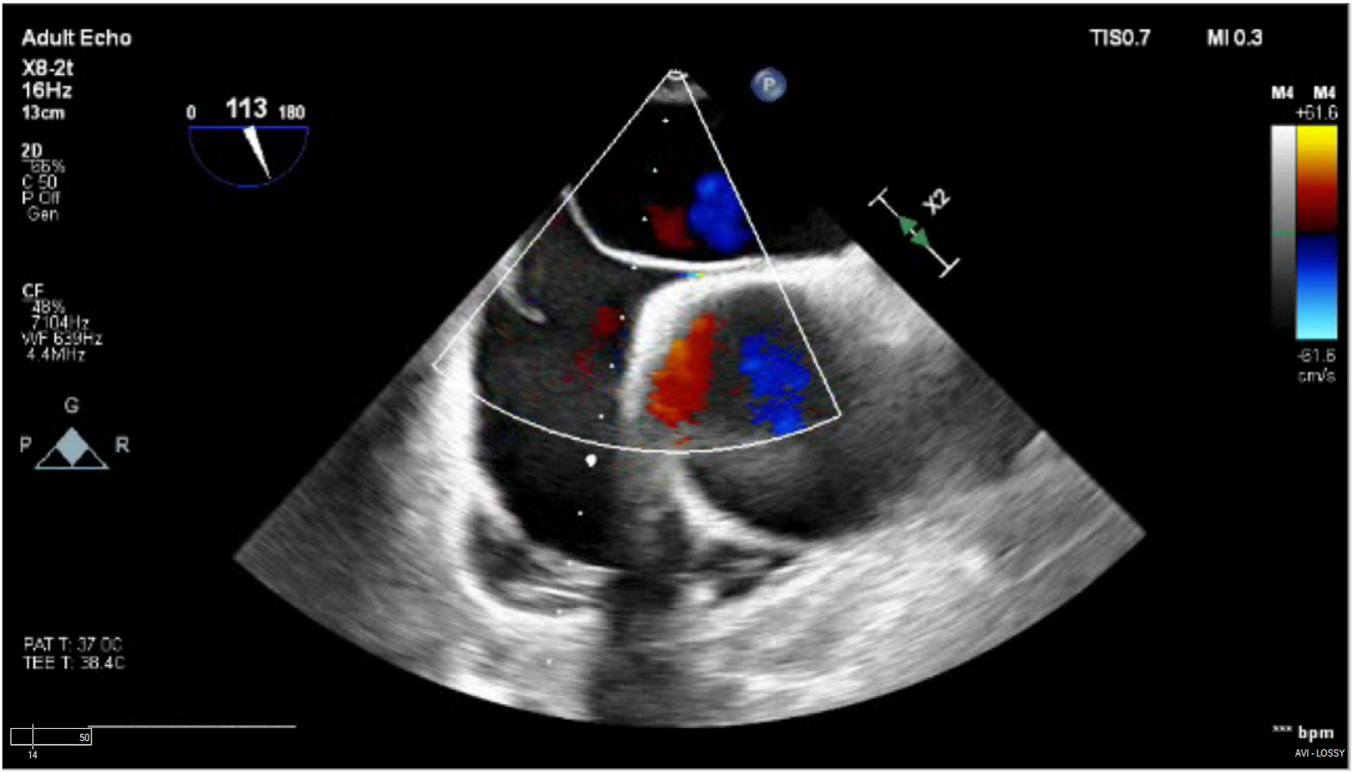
Transoperative transesophageal echocardiogram conducted before the commencement of the Bentall procedure, depicting a short-tunneled patent foramen ovale with minimal interatrial flow.
